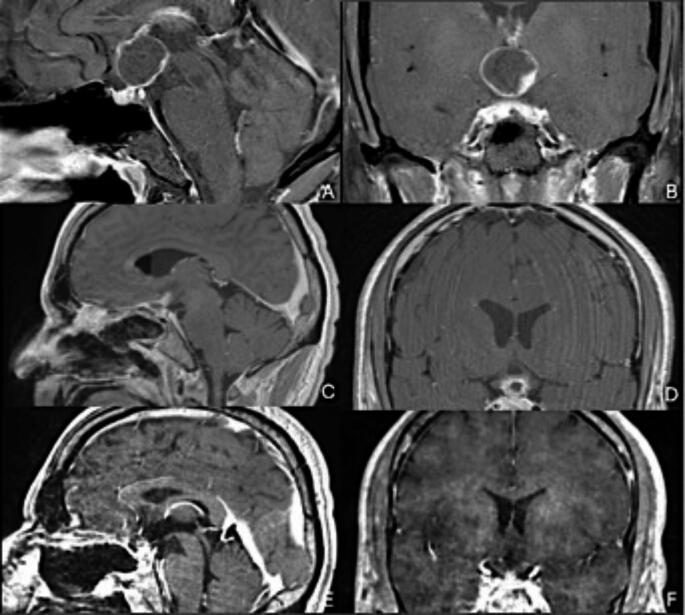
Panel A-B show preoperative MRI with a complex solid-cystic suprasellar lesion without calcification. Panel C-D show the immediate postoperative MRI demonstrating decompression of the cystic component of the tumor with residual enhancing tumor along the optic chiasm following endoscopic endonasal surgery for decompression and diagnosis. Panel E-F show an MRI at most recent follow up after treatment with combined BRAF/MEK inhibitor therapy with minimal residual enhancement, which could be gliotic tissue versus miniscule residual nodular component of the tumor
